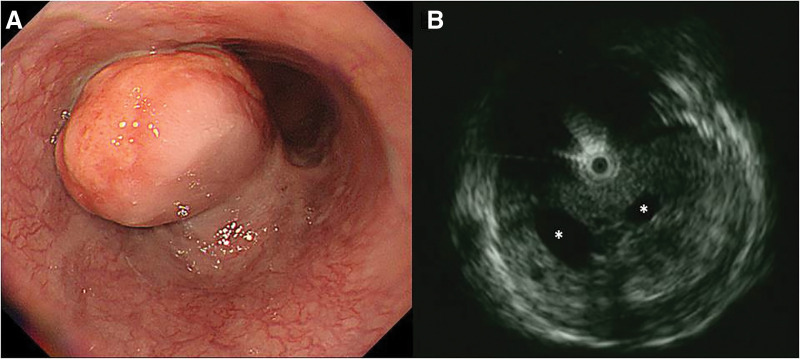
(A) An endoscopic examination identified a submucosal tumor in the lower esophagus, approximately 40 cm from the incisors. (B) EUS revealed a hypoechoic mass with multiple cystic structures (asterisks). EUS, endoscopic ultrasonography
